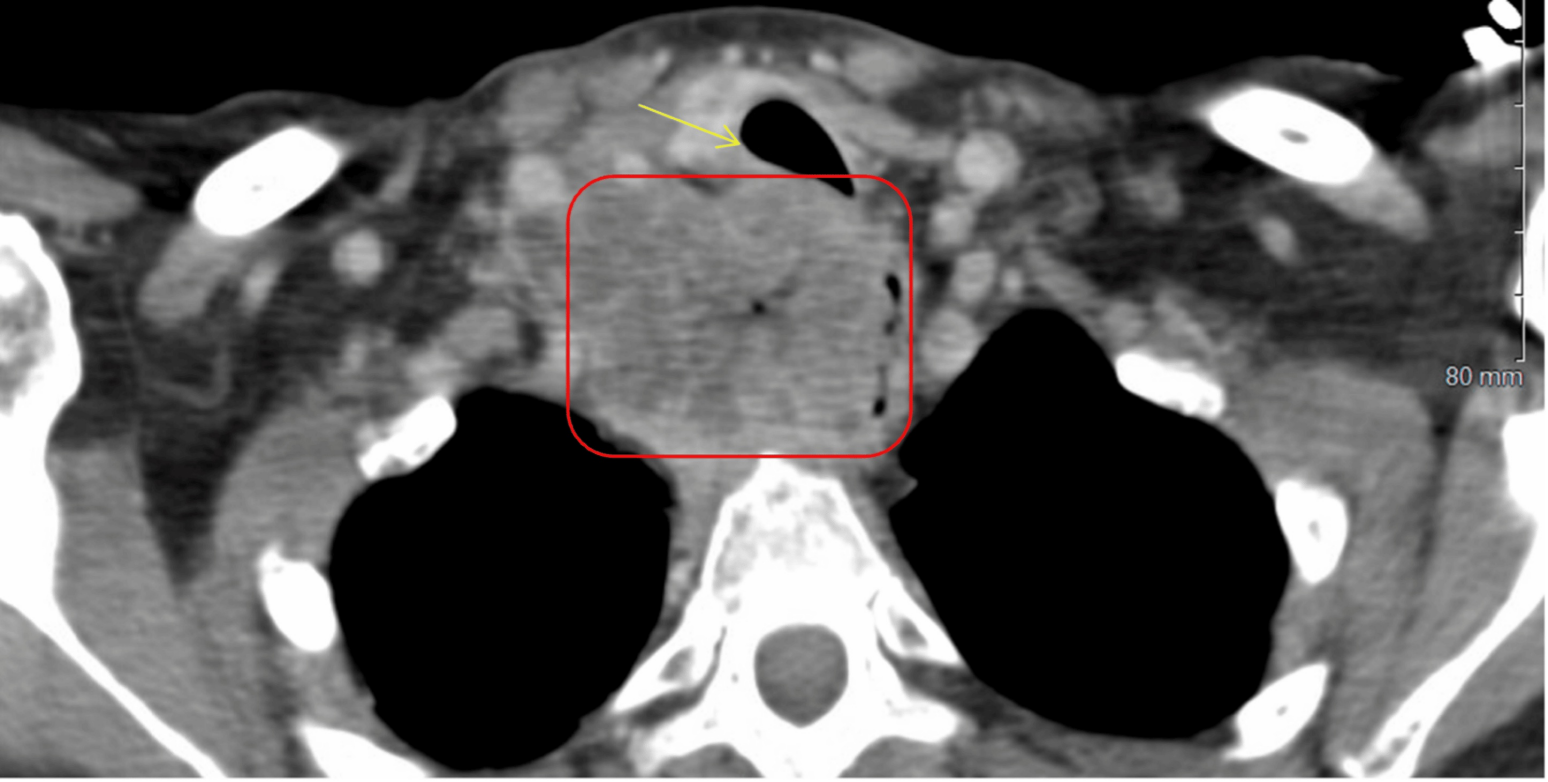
The CTA pulmonary (axial view) shows a large right paratracheal mass (red box), with an impression on the trachea and esophagus, with a deviation to the left (yellow arrow). There is a tracheal invasion within the posterior midsegment with approximately 70% stenosis of the trachea at this point. CTA: computed tomographic angiography
